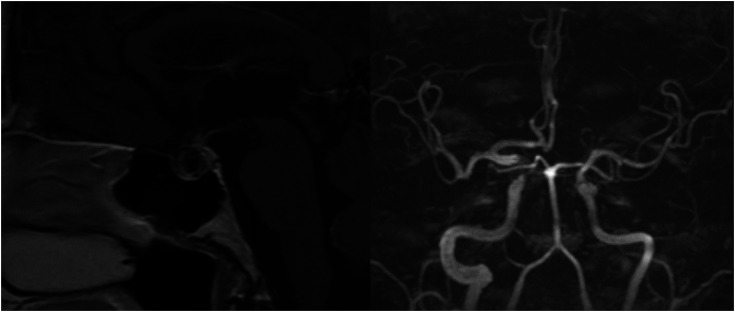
Follow-up magnetic resonance imaging (MRI) 1 year after treatment. The image on the left shows occlusion of the aneurysm and partial empty sella with substantially decreased pituitary volume. The image on the right shows a time of flight MRI with an absence of flow in most of the aneurysm sac and minor residual flow in the region of the aneurysm neck; the low signal flow in the segment of the internal carotid artery where the stent is placed is due to artifacts.
